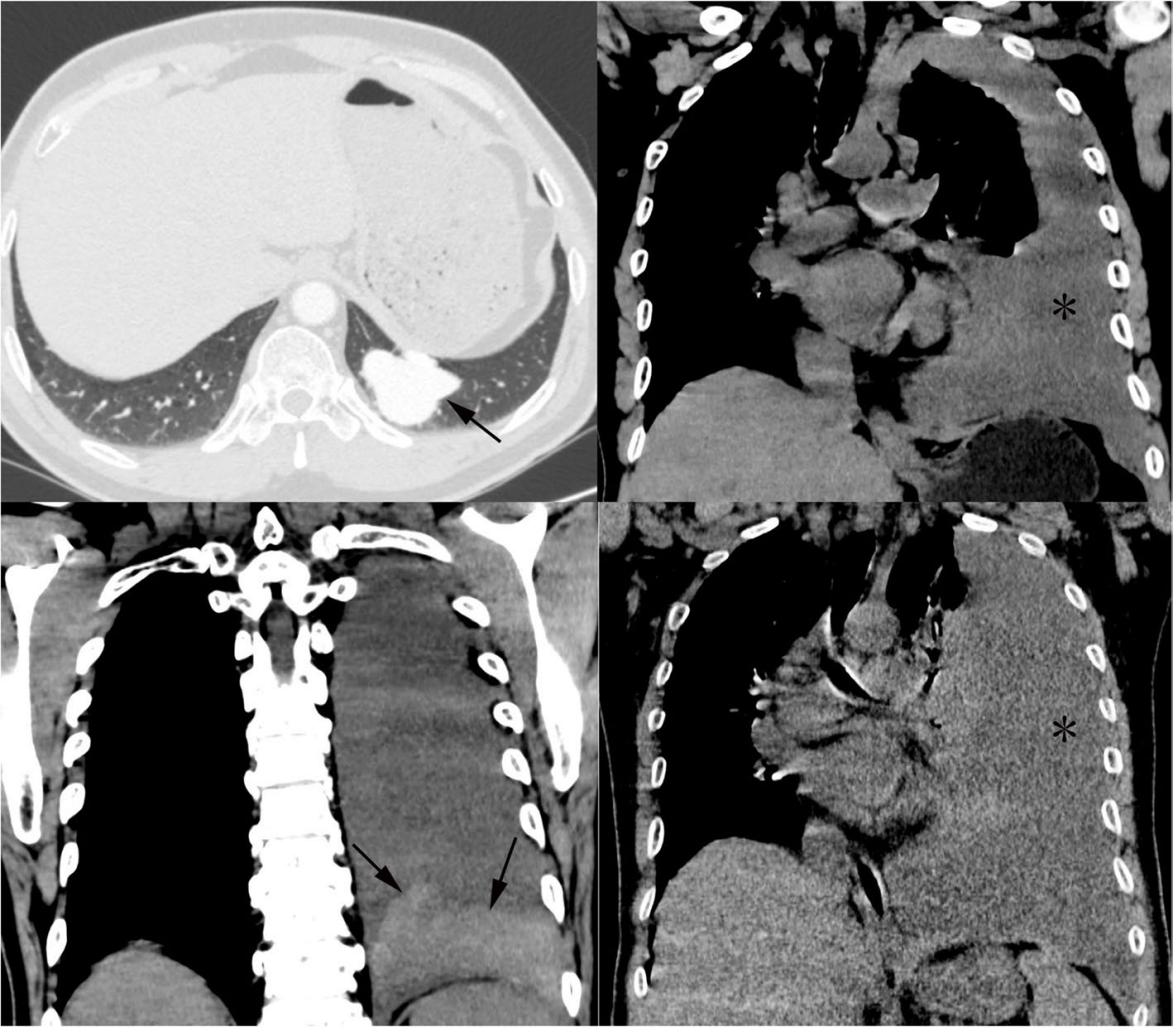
A Contrast-enhanced CT revealed PAVM with the formation of aneurysm 18 months previously. B-C Chest unenhanced CT indicated the left pleural effusion together with irregular patchy high-dense opacity at the upper aspect of the diaphragm. D Chest CT at the interval of six days showed a prominent increase in the amount of left pleural effusion
